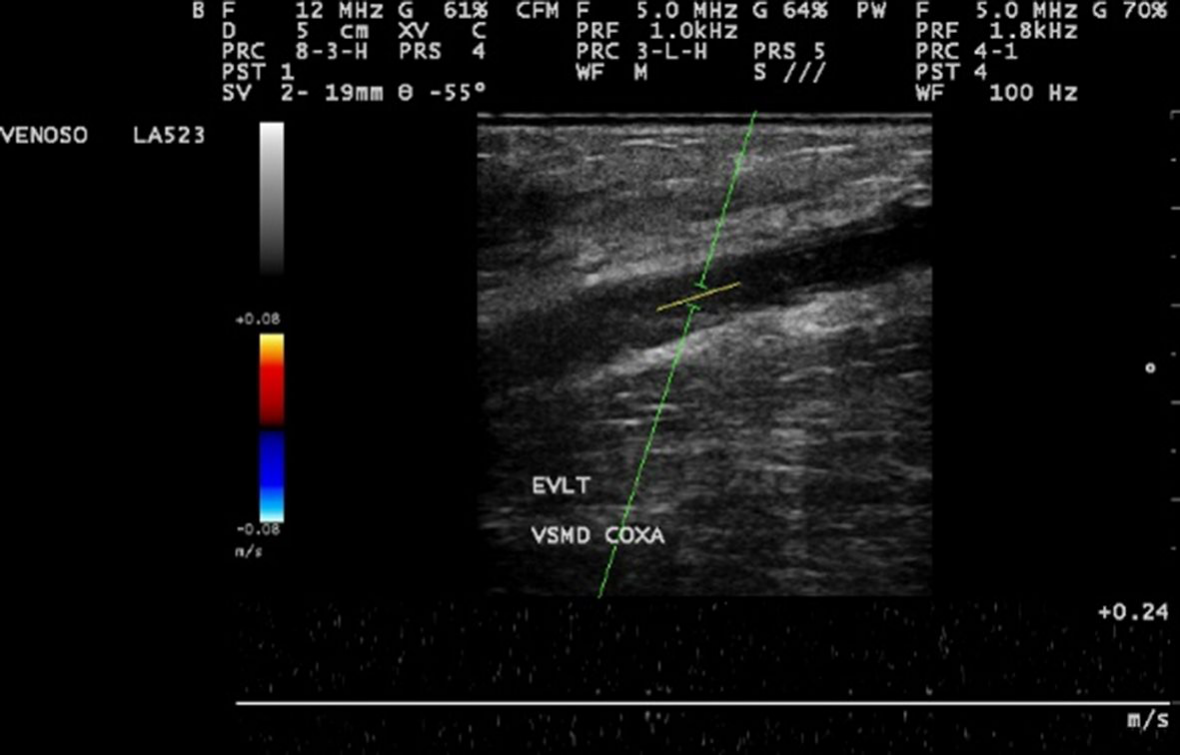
Control ultrasound examination at 7 postoperative days, demonstrating absence of flow in the saphenous vein.
